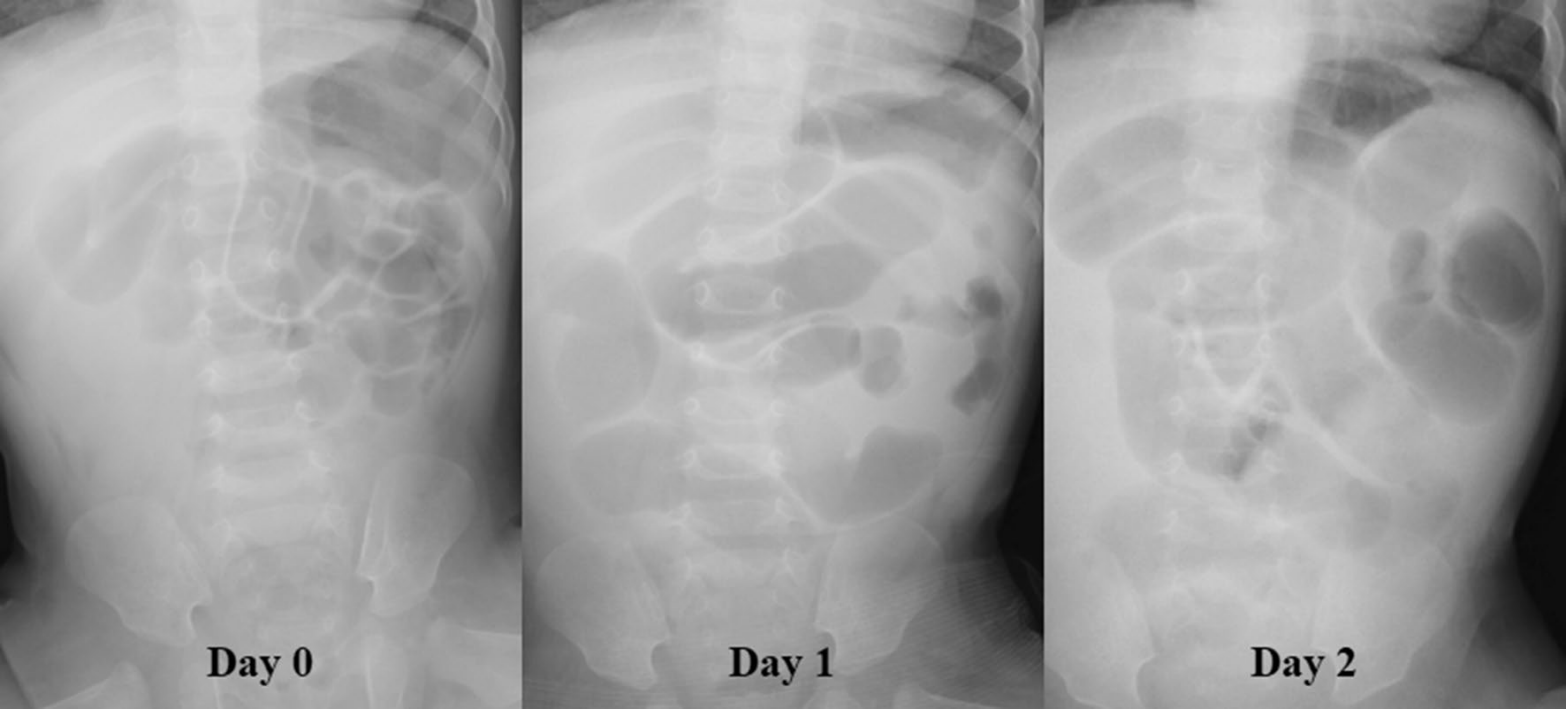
Abdominal X-ray findings
